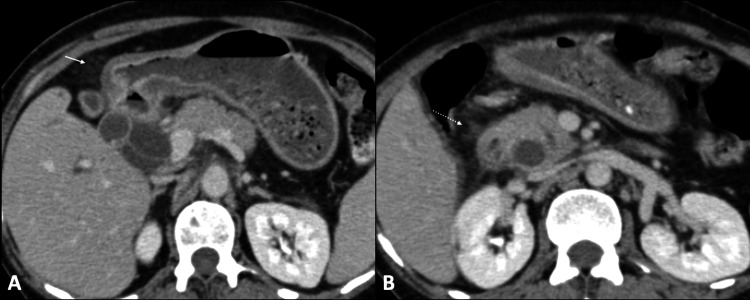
Peritoneal spread Axial sections of the CECT of the abdomen demonstrate stranding of gastrocolic (white arrow in Figure 2A) and hepatoduodenal (white arrow in Figure 2B) ligaments. CECT: contrast-enhanced computed tomography
